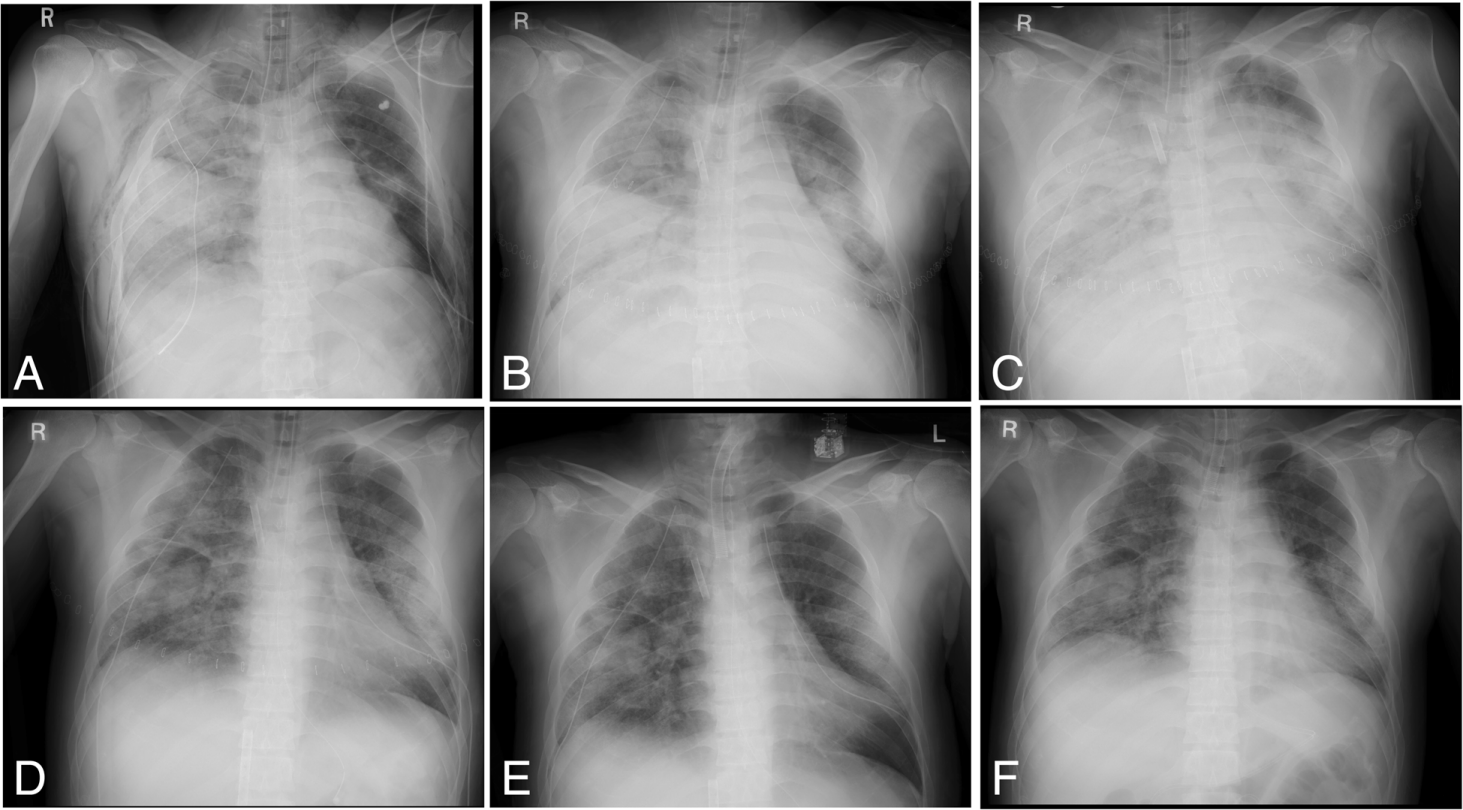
Chest X-ray images during the course of treatment: a initial; b postthoracotomy; c day 3 under full-heparin ECMO; d day 8 after heparin-free ECMO; e day 10 after removing ECMO; f day 15 after removing thoracic tubes
